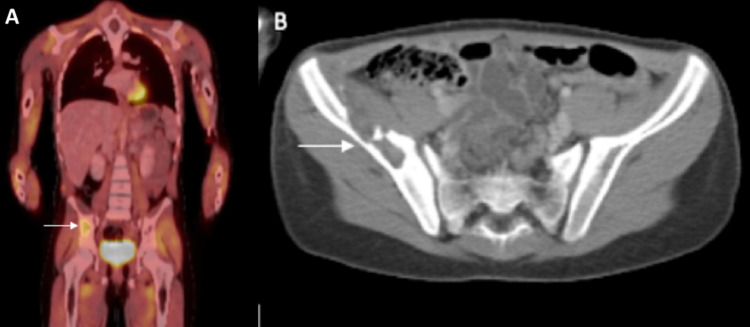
8-year-old female presenting with hip and lower extremity pain (Case 14). (A) AP PET/CT demonstrating hyperintensity of the right ilium. (B) Axial CT demonstrating an osteolytic lesion at the right ilium.
